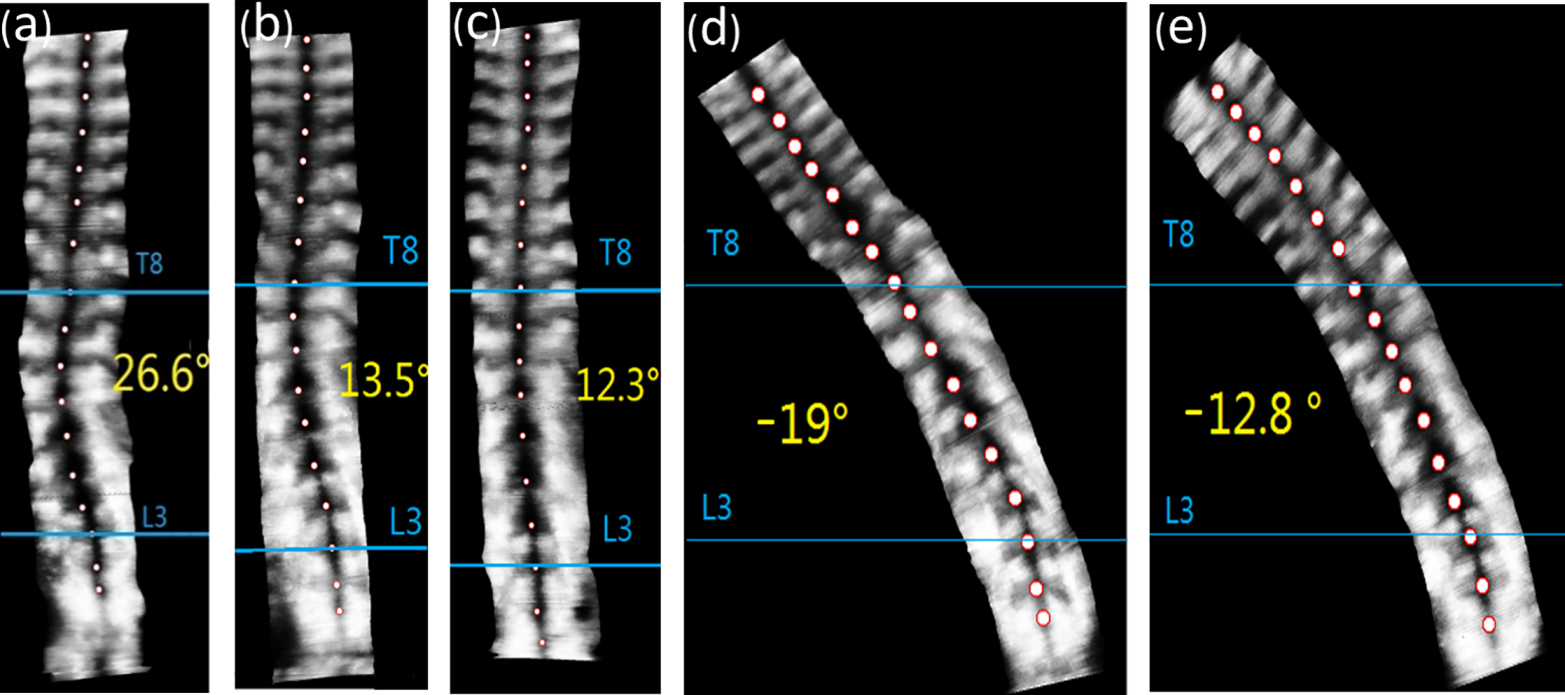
Ultrasound images in (a) standing position (b) supine position (c) prone position (d) sitting with lateral bending position (e) prone with lateral bending position. The left thoracolumbar curve ranged from T8 to L3 (apex at T11) with the magnitude of 26.6° in standing position, 13.5° in supine position, 12.3° in prone position, -19° in sitting with lateral bending position, and -12.8° in prone with lateral bending position (negative value refers to the curve being corrected to the opposite direction).
